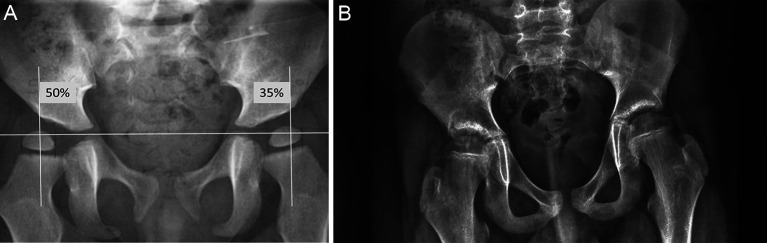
(A) Preoperative radiograph of a 3-year-old patient with spastic quadriplegia (GMFCS III) and hip subluxation with Right MP 50% and Left MP 35%. (B) Ten years after isolated STR showing good outcome.
